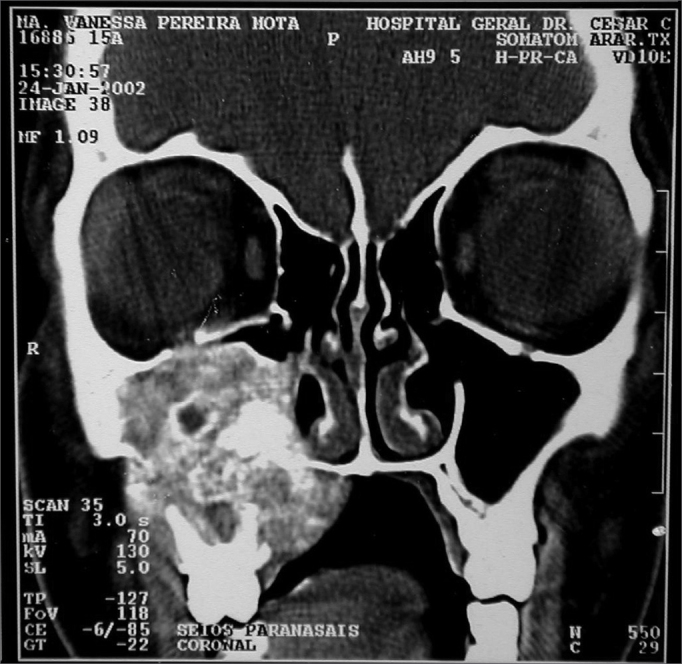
CT scan - coronal view of a cement-ossifying fibroma (case 6).
